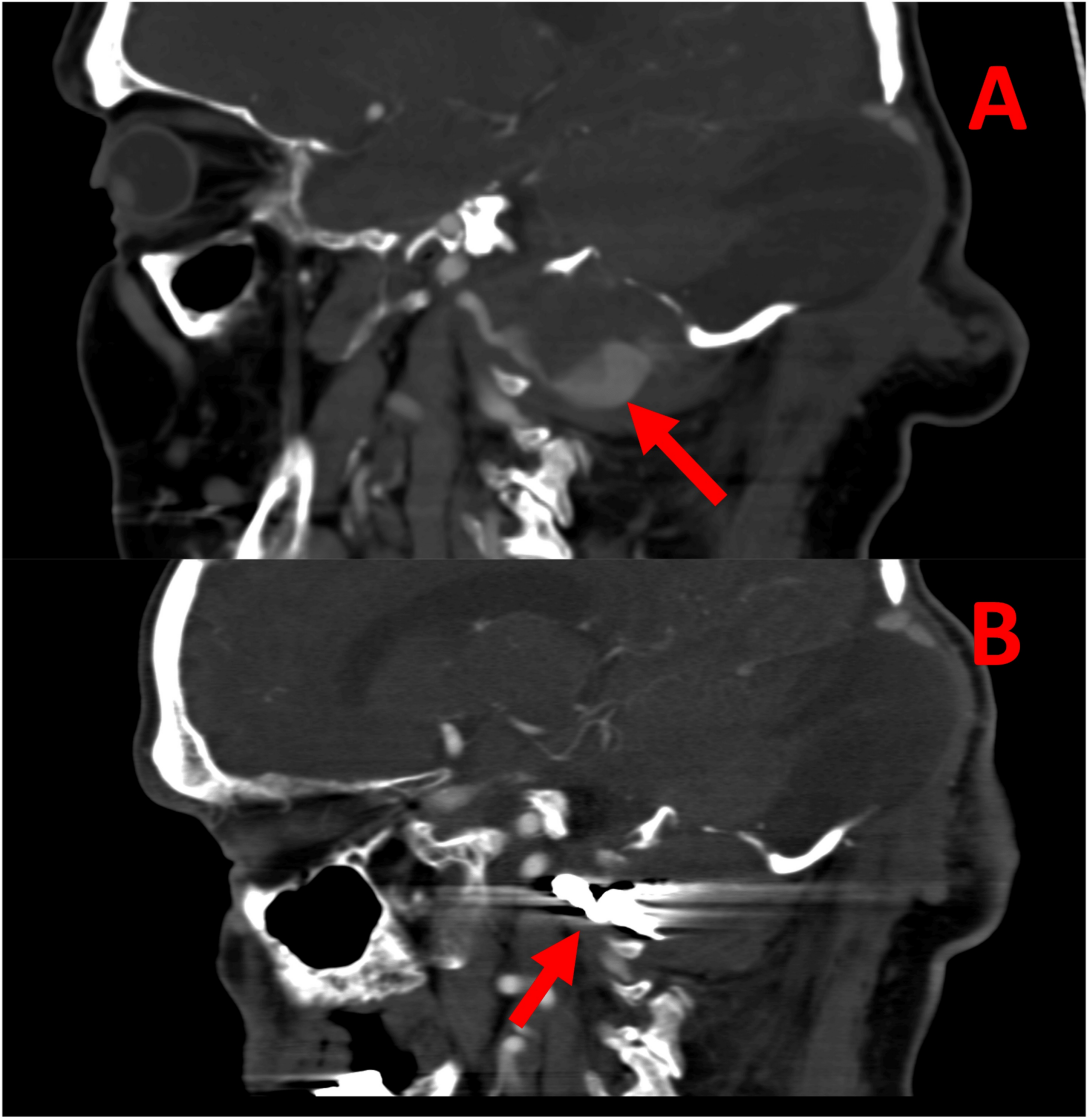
Computed tomography angiography of the patient's right vertebral pseudoaneurysm - pre-treatment and 11 months post-treatment. (A) Pre-treatment sagittal section: Contrast leaking into the culprit pseudoaneurysm from the right vertebral artery (red arrow). (B) Post-treatment sagittal section: Coils are present in the pseudoaneurysm neck and proximal right vertebral artery (red arrow) and no filling is seen in the pseudoaneurysm.
